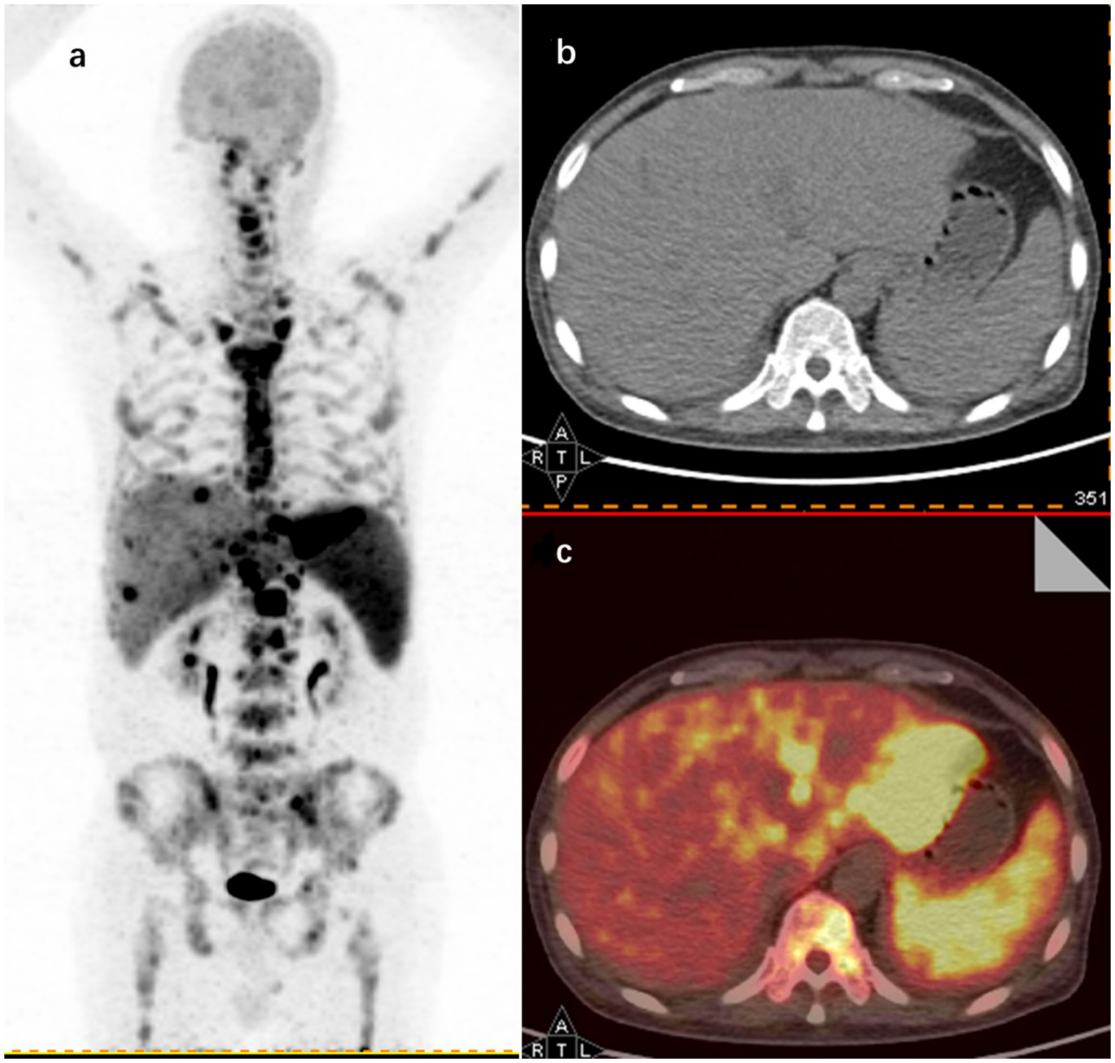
18F-FDG PET/CT findings in a 62-year-old male FUO patient with a final diagnosis of lymphoma. (A) Maximum intensity projection (MIP) images of whole-body 18F-FDG PET coronal sections show hypermetabolic sites in the left hepatic lobe (SUVmax 23.1), spleen (SUVmax 8.5), and throughout the skeleton (SUVmax 16.8). Representative axial sections of CT (B) and image fusion (C) show increased uptake in the left hepatic lobe and spleen.
